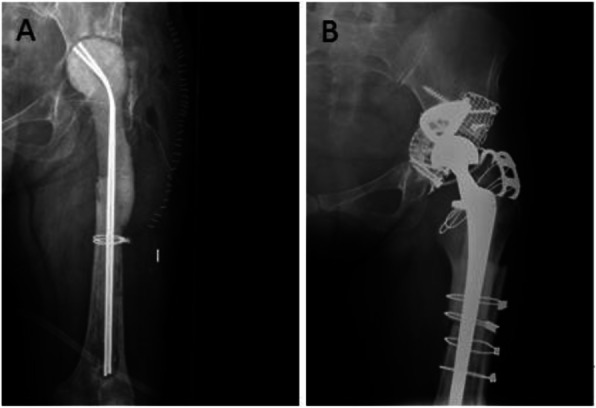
a-b. Preoperative radiograph (first stage) in a patient with septic loosening of the acetabular component and the last radiograph after reconstruction (case 2)
